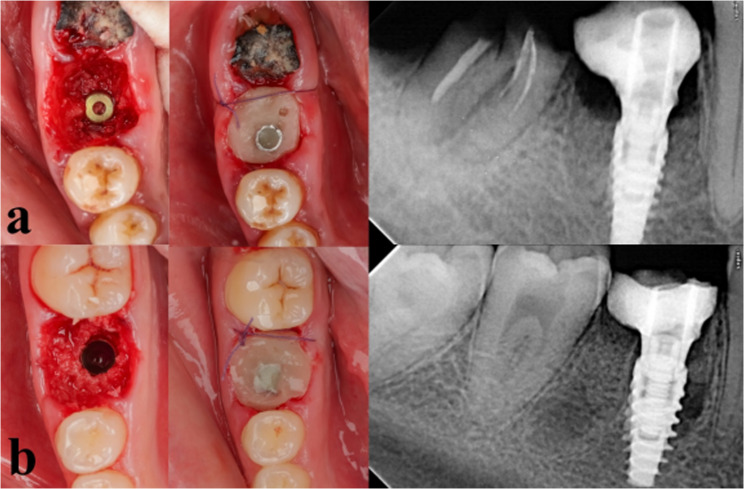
Displays jumping gap filling with allograft bone and sealing the sockets with custom made healing abutment and periapical xray immediate postoperative
